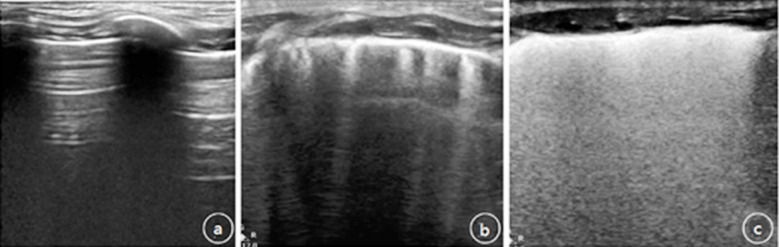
Lung ultrasound results of babies in two groups.
